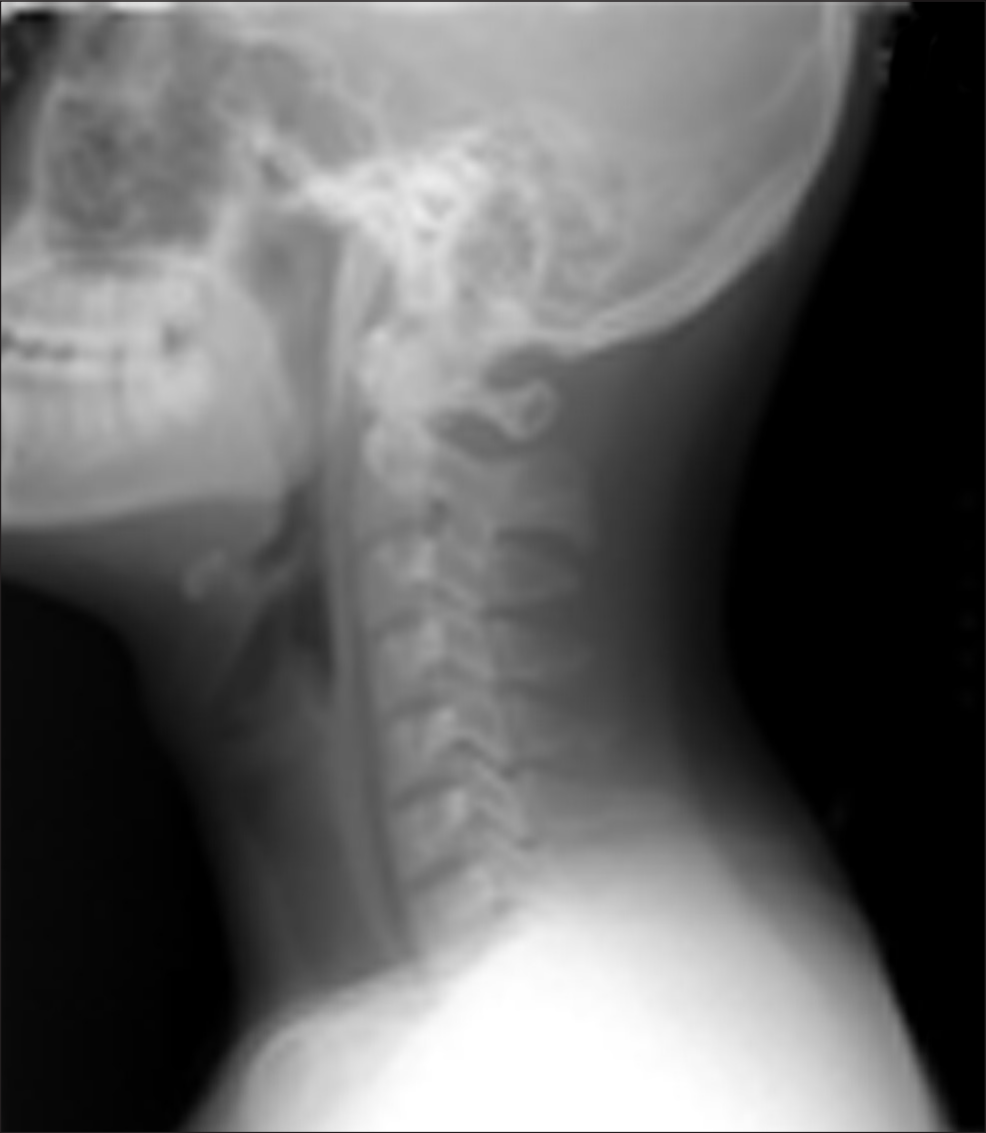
Lateral neck X-ray case 1 reveals emphysema in the prevertebral space
